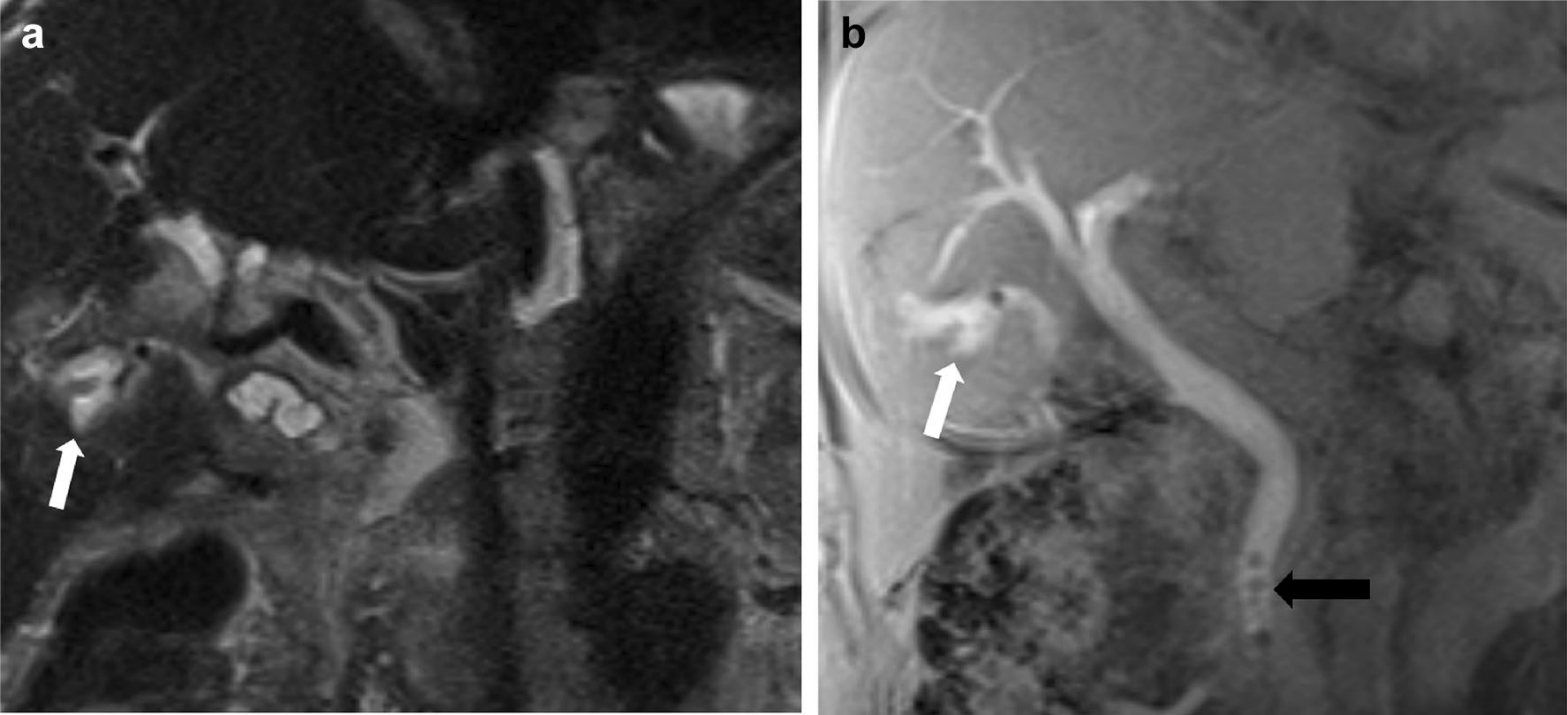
An 84-year-old male status post laparoscopic cholecystectomy for gallstone pancreatitis, with postoperative bile leak, confirmed using Eovist. A strong suspicion of a leak from the cystic duct stump was raised, which was confirmed on ERCP. a and b Coronal T2W image and 90 min delayed post-Eovist T1W image, respectively, showing a collection in gallbladder fossa with excretion of Eovist into the collection consistent with bile leak (white arrows). Pneumobilia in the terminal CBD (black arrow in image B) was better seen in a CT study performed earlier (not shown)
